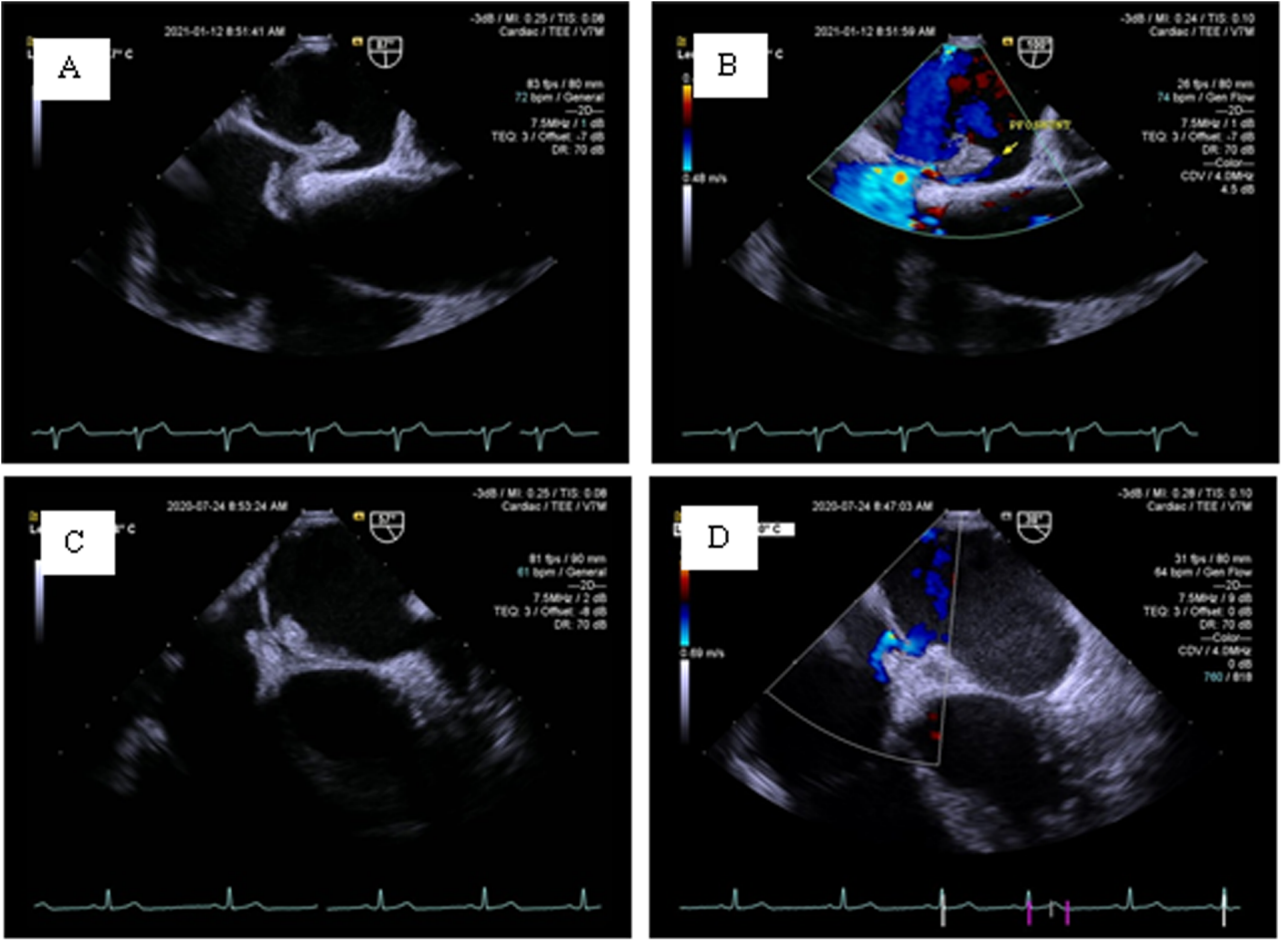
TEE examination of medium to substantial rRLS. (A,B) A large PFO (4.5 mm) with a long tunnel (13.5 mm) was implanted with a 30/30 mm PFO occluder. The left disc was degraded to 8 mm and did not completely cover the left side of the PFO. A tunnel-like channel was formed in the occluder. (C,D) A PFO with a small ASD; the device achieved closure of the PFO and retained the small ASD. On 2D-TEE, the small ASD was not clearly displayed. Color Doppler flow imaging showed a transseptal shunt through the small ASD.
